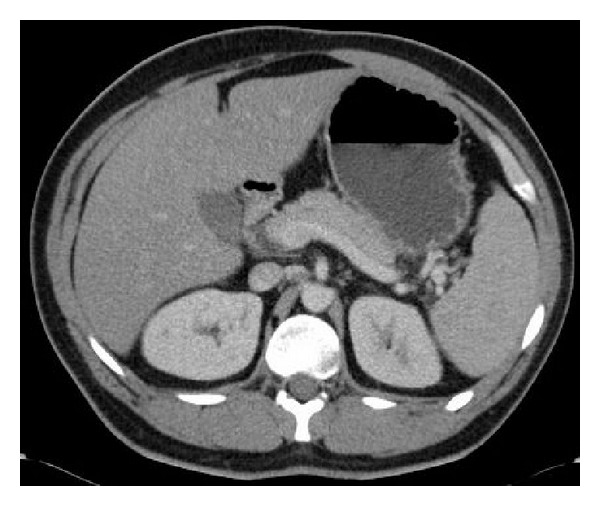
A coronal section of the contrast computed tomography scan, revealing a fatty liver and normal pancreas.
